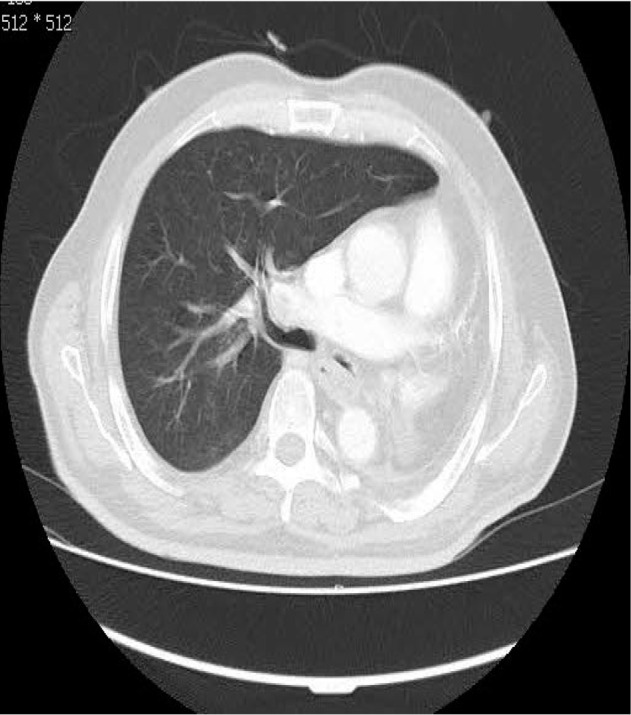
Axial lung window CT scan, anatomic deviation after left pneumonectomy
